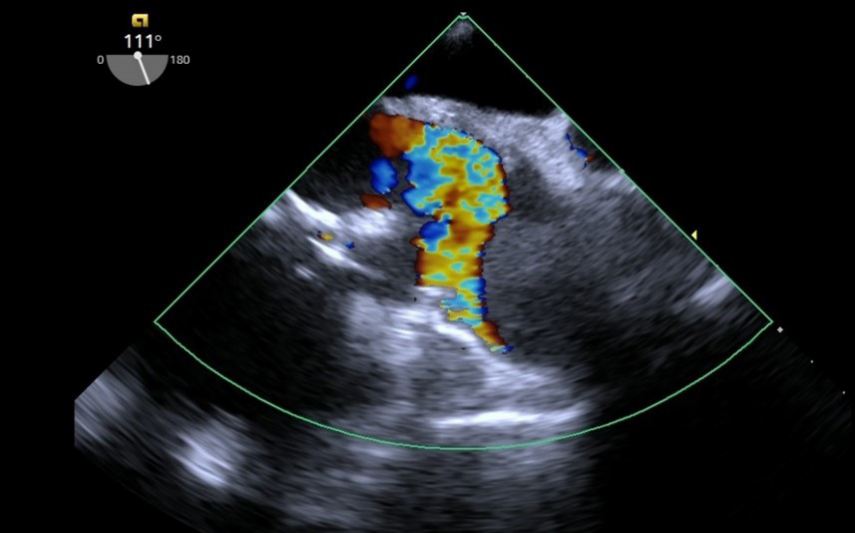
Transesophageal Doppler echocardiography showing the subocclusive
